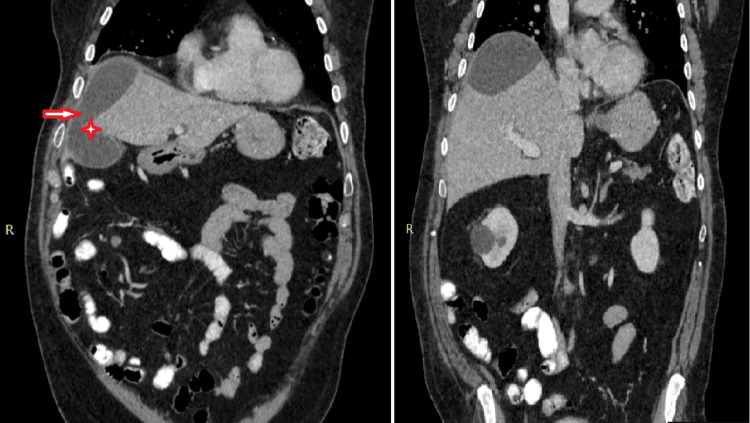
Coronal plane of the abdominal CT The star highlights the continuity between the gallbladder and the subcapsular lesion.
